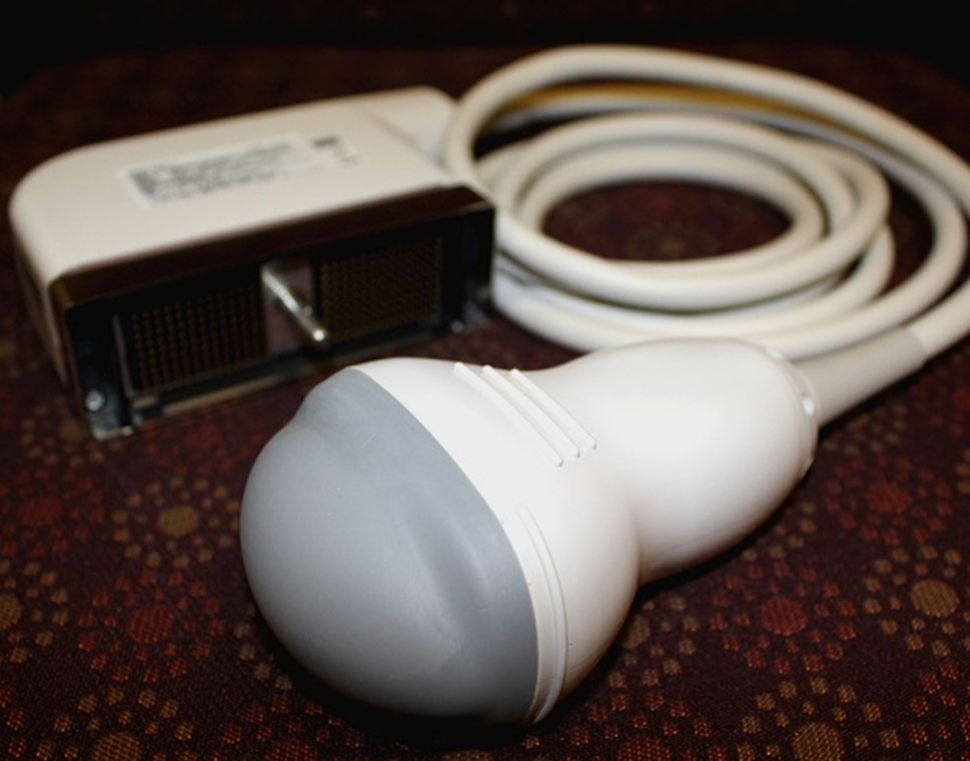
Fukuda Denshi 3D/4D ultrasound probe
